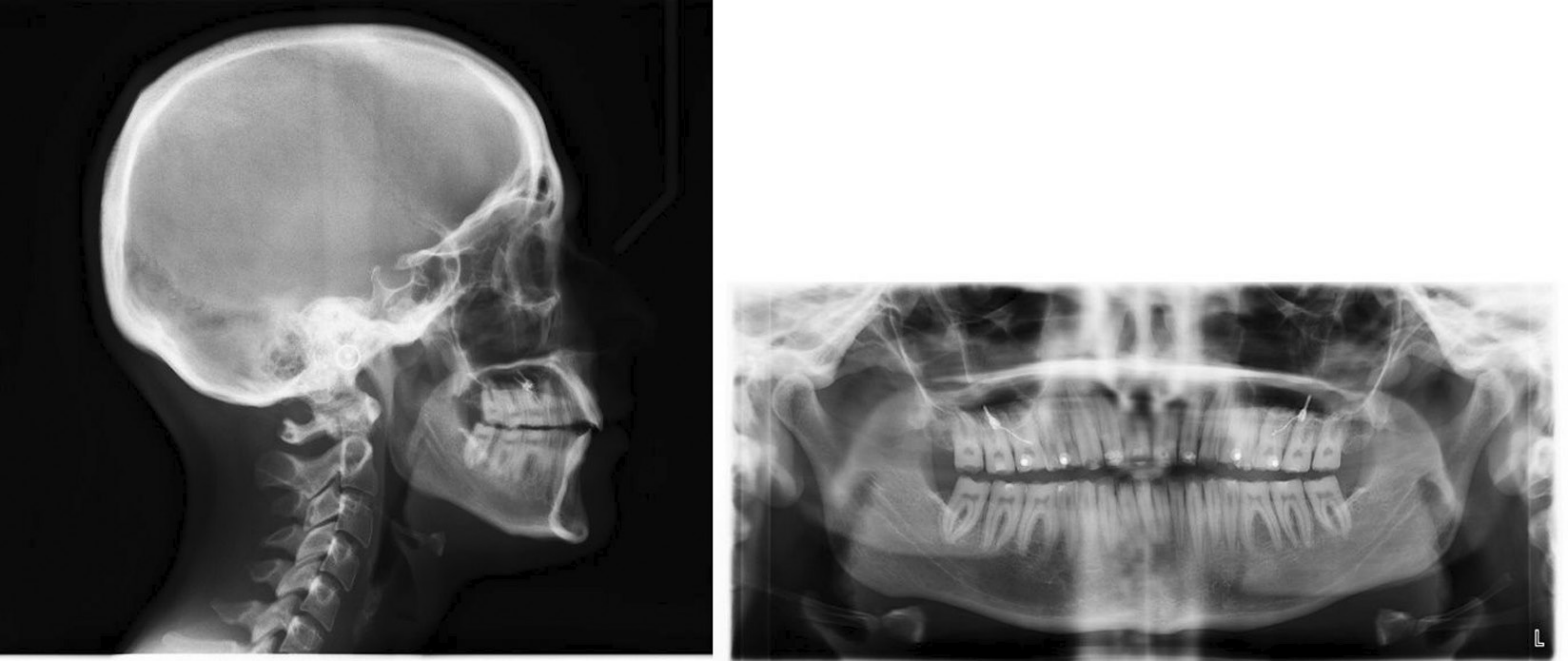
Post‐treatment radiographs
